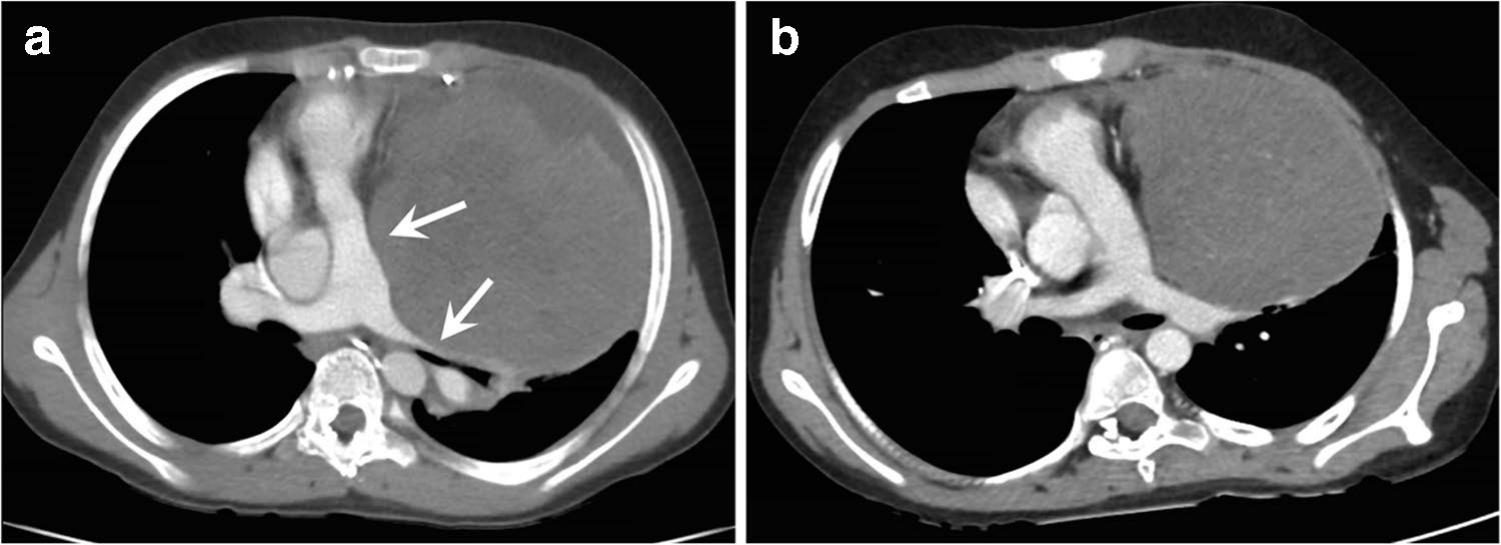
A 13-year-old boy with an anterior mediastinal mass (non-Hodgkin B lymphoma). The patient presented to the emergency department with malaise and dyspnea. A large anterior mediastinal mass was seen on axial contrast-enhanced chest computed tomography (CT) (a). Compression with severe stenosis of the left pulmonary artery was seen (*arrows*). b Follow-up axial contrast-enhanced CT after chemotherapy 4 weeks later shows significant volume reduction of the mass and resolution of the pulmonary artery compression
